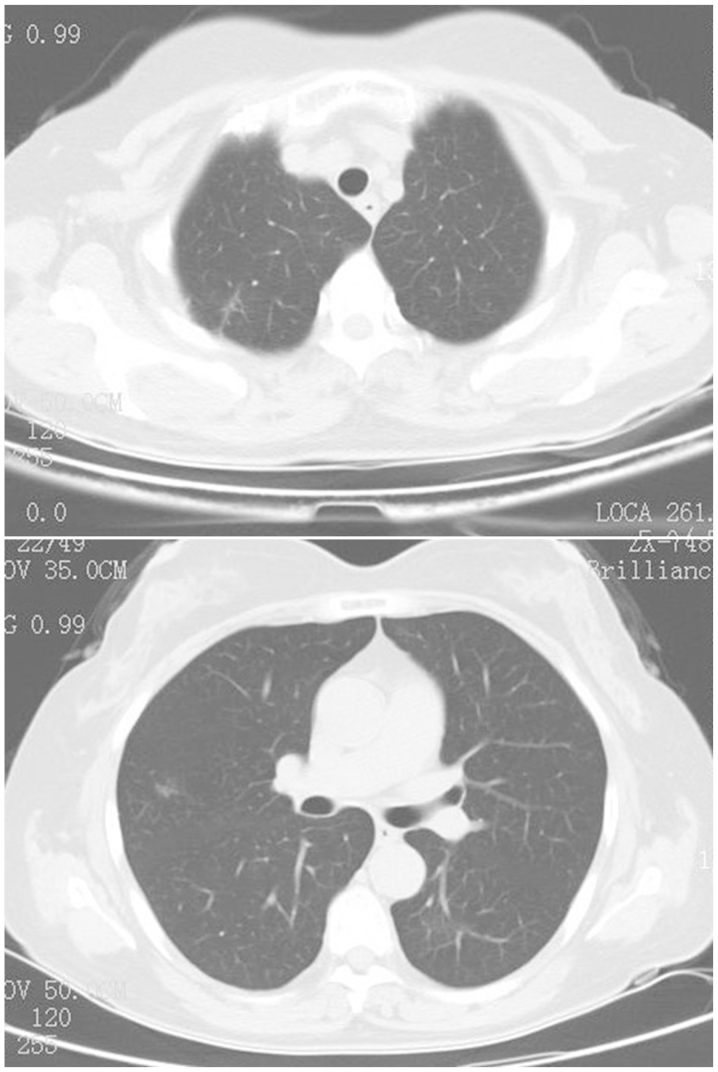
Chest radiography six months post-treatment showing a complete resolution of the previously observed bilateral patchy, nodular consolidations in the two lungs.
